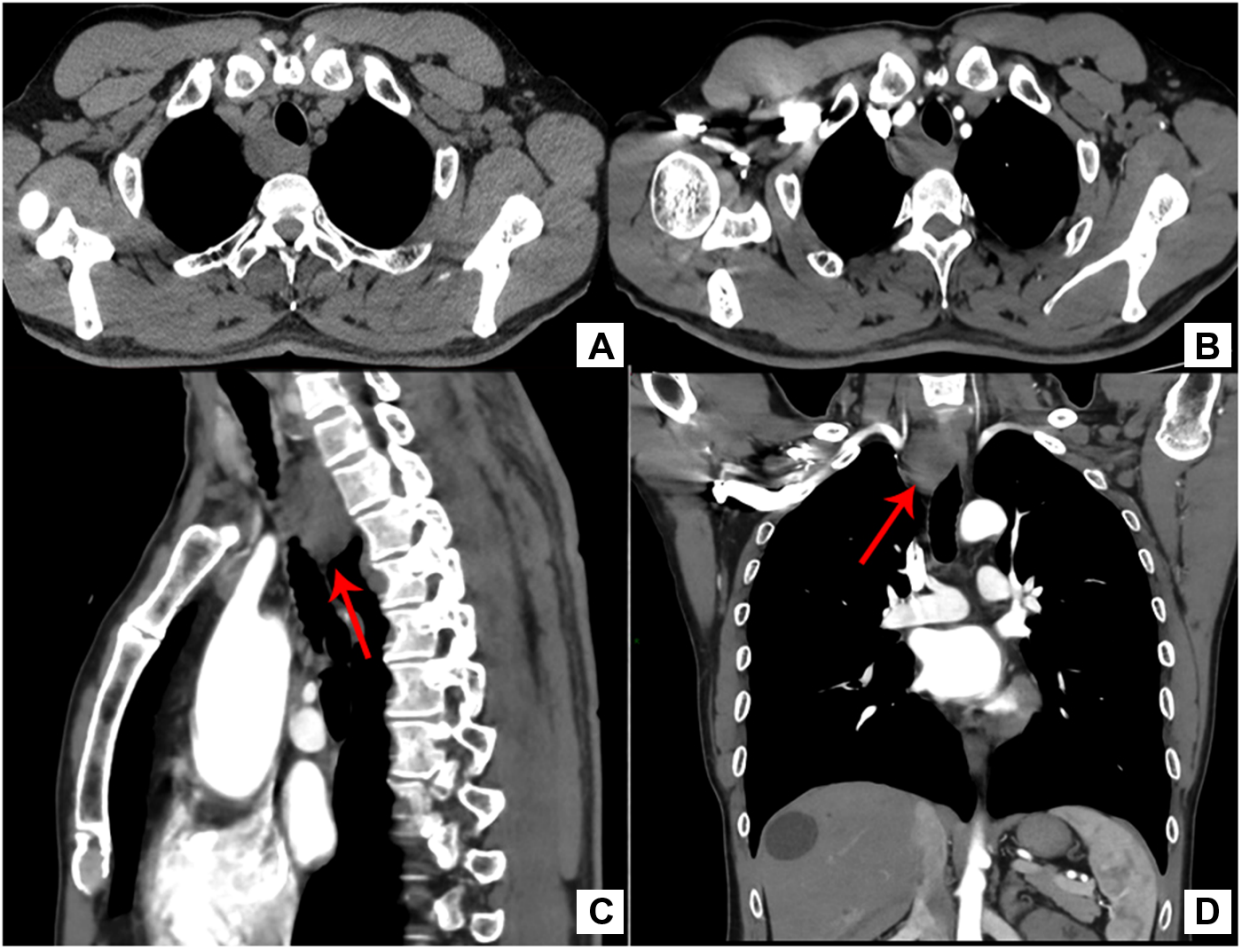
(A) CT revealed a mass on the right side of the upper and posterior mediastinum and the adjacent trachea and esophagus were pushed. ((B) Transverse, (C) sagittal, (D) Coronal) contrast-enhanced CT scan showed an unobvious enhanced mass with clear tumor boundaries.
